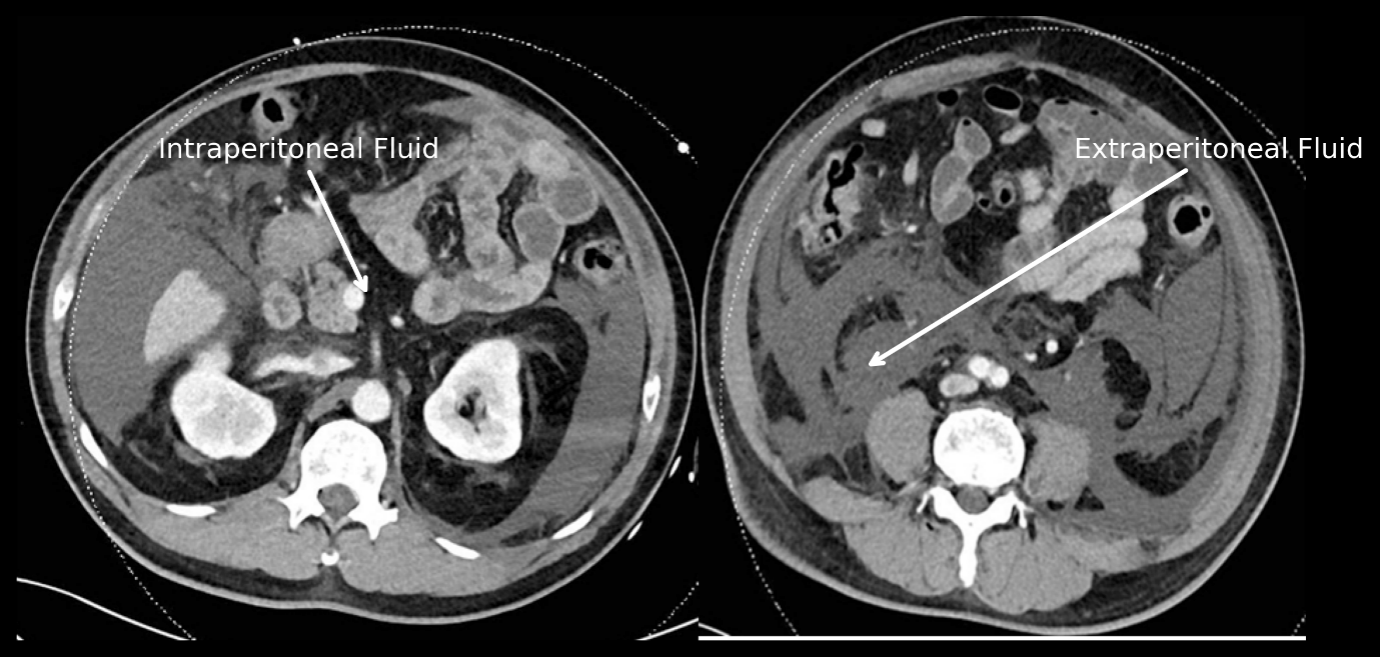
Abdominal CT done postoperatively on ICU admission showing fluid seepage into Extraperitoneal and Intraperitoneal Compartments. The left panel demonstrates intraperitoneal fluid (white arrow on the left) around the mesentery and bowel loops, while the right shows extraperitoneal fluid (white arrows on the right) in the retroperitoneal and perivesical spaces.
